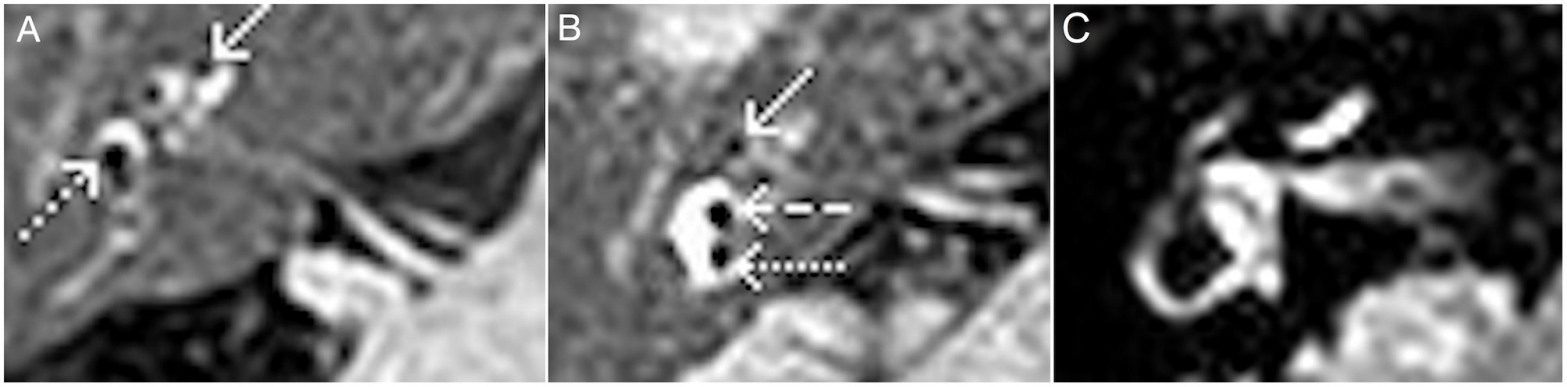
MRI (inversed recovery) of a right inner ear, axial plane. (A) Evidence of cochlear (arrow) and vestibular hydrops (dotted arrow). Endolymphatic space is clearly visible by its hypointense (dark) signal, compared to the hyperintense (bright) signal of the contrast-enhanced perilymphatic space. (B) Evidence of cochlear (arrow) and vestibular hydrops; saccule (dashed arrow) and utricle (dotted arrow). (C) Regular contrast enhanced perilymphatic space of vestibule and cochlea; no enlargement of endolymphatic space.
